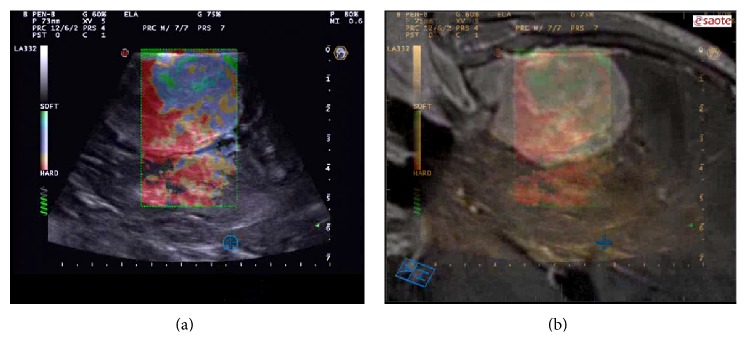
Elastosonography imaging. In the left box is visualized the elastosonogram (strain elastography) of the lesion; in the right box, it is fused with corresponding preoperative MRI. Elastosonography shows that the tumor has two different consistencies; this finding was confirmed by surgeon feelings. Deeper into the lesion, temporal lobe has higher stiffness if compared with normal findings; this is due to mechanical compression from meningioma.
